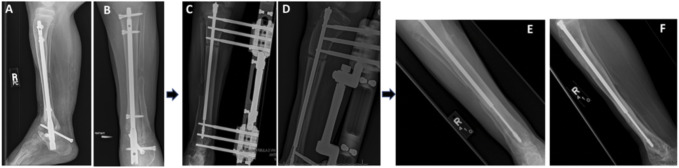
Patient right tibia and ankle radiographs demonstrating timeline of infection. A, Lateral and (B) AP radiographs at the time of *M. morganii* infection demonstrating persistent irregularity to the distal right tibia and an osteotomy gap but with no lucent lesions or signs of osteomyelitis. C, AP and (D) oblique radiographs 1.4 years after *M. morganii* infection. E, AP and (F) lateral radiographs 2.5 years after *M. morganii* during our patient's methicillin-sensitive *S. aureus* (MSSA) infection demonstrating lucency of distal tibia.
